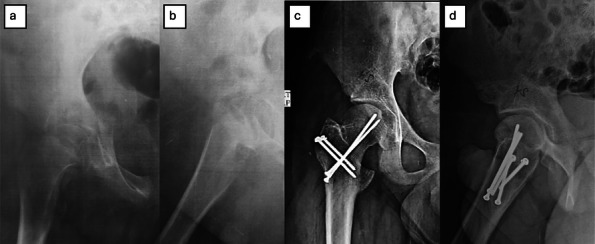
a, b Preoperative radiographs (ap and lateral) showing right SCFE in 10-year-old boy. c, d Postoperative radiographs (ap and lateral) at the final follow-up visit (7 year) and after fixation by by two cannulated screws
